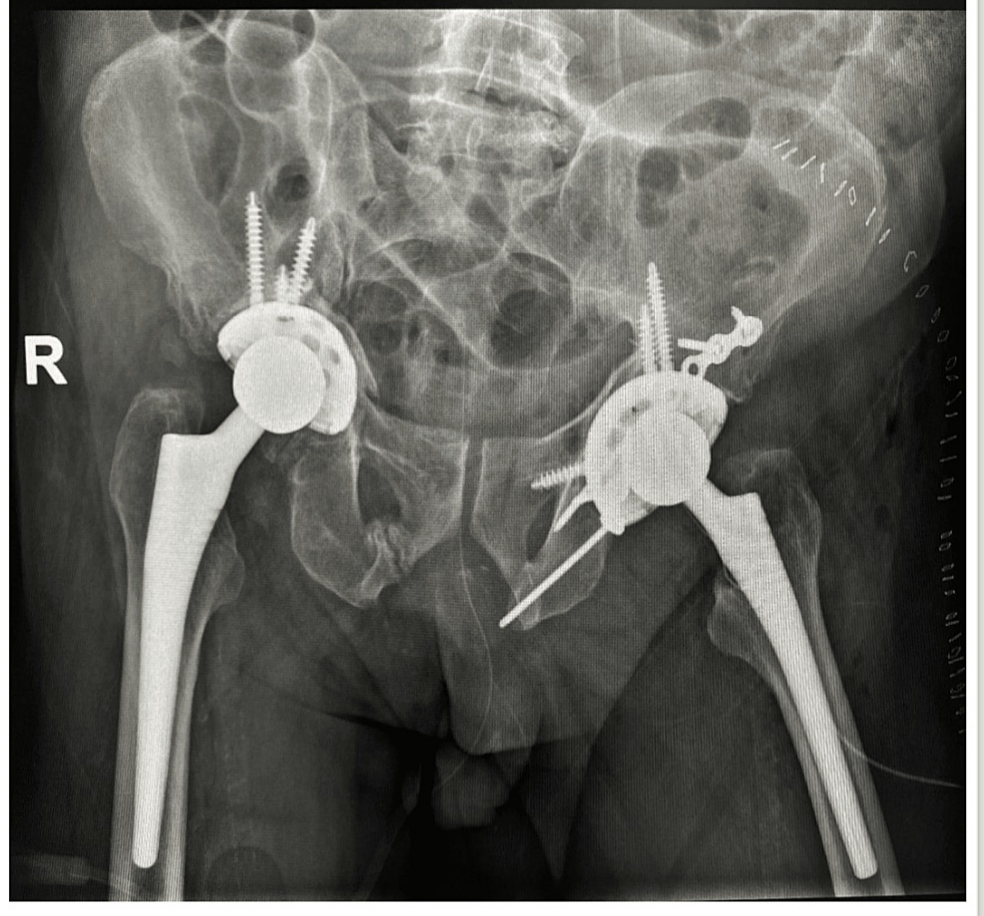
Post-operative x-ray of the pelvis showing left hip ORIF and THA. ORIF: Open Reduction Internal Fixation, THA: Total Hip Arthroplasty.
